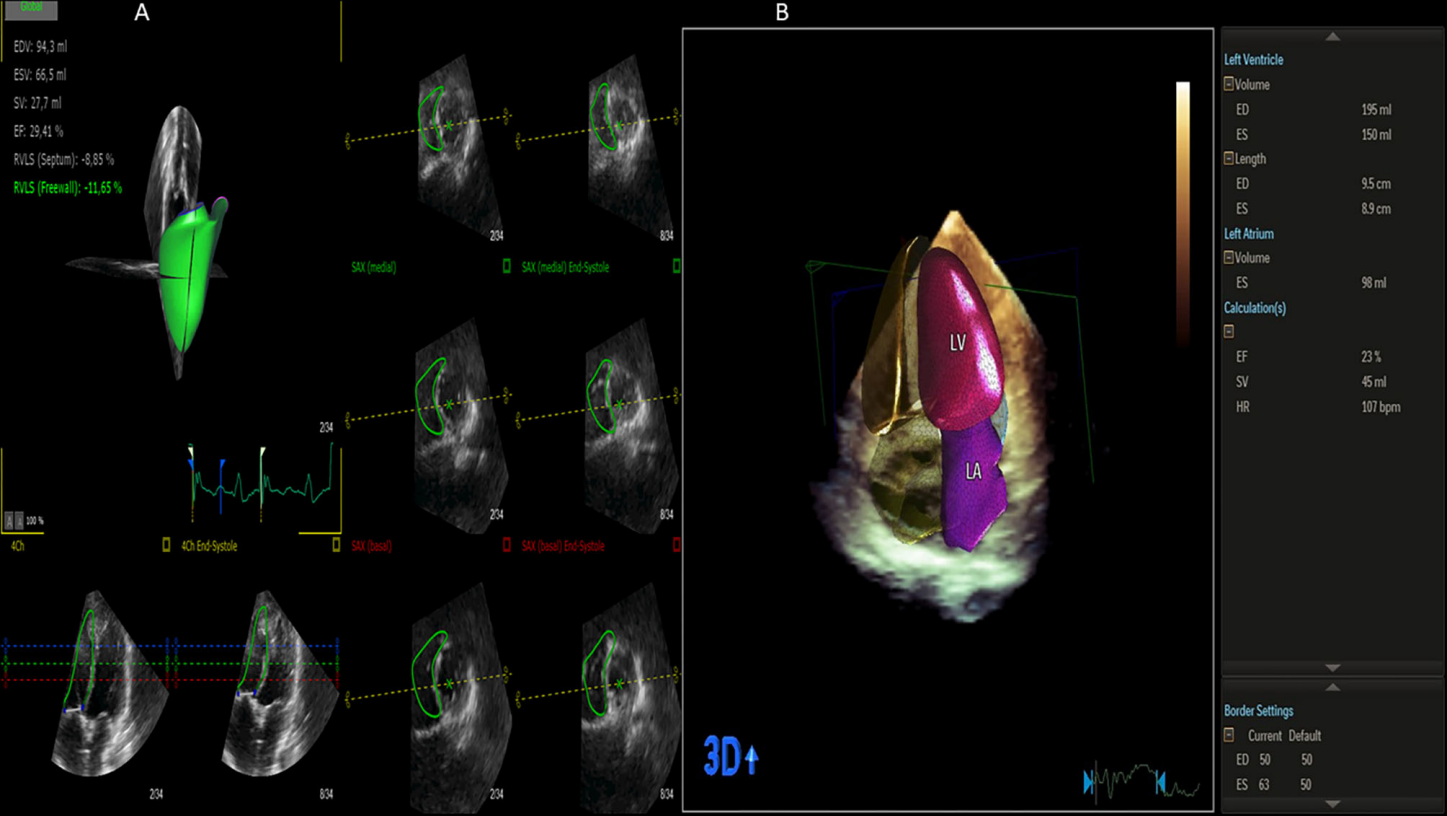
Left and right ventricle analysis by 3D echocardiography (artificial intelligence). (A) Right ventricle analysis. (B) Left ventricle and left atrium analysis.
